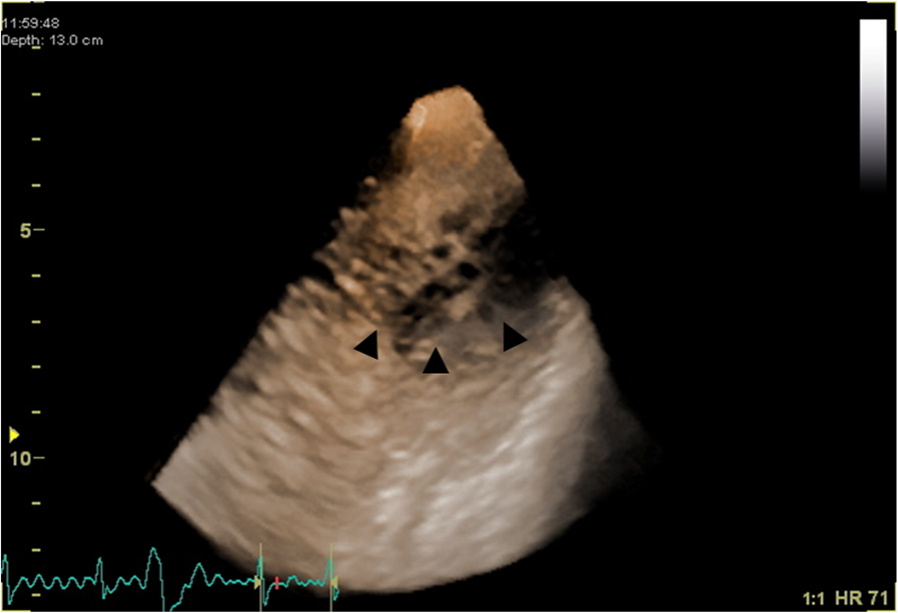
Real-time three-dimensional echocardiography demonstrated the ‘honeycomb appearance’ in the apical lateral wall (arrowheads) in our patient (Case 2).
